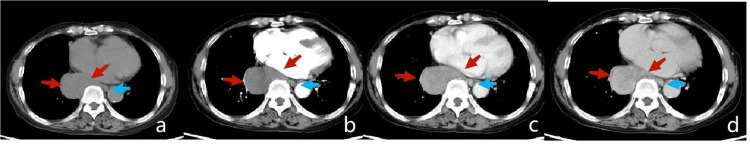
Plain CT and enhanced scan of the chest
